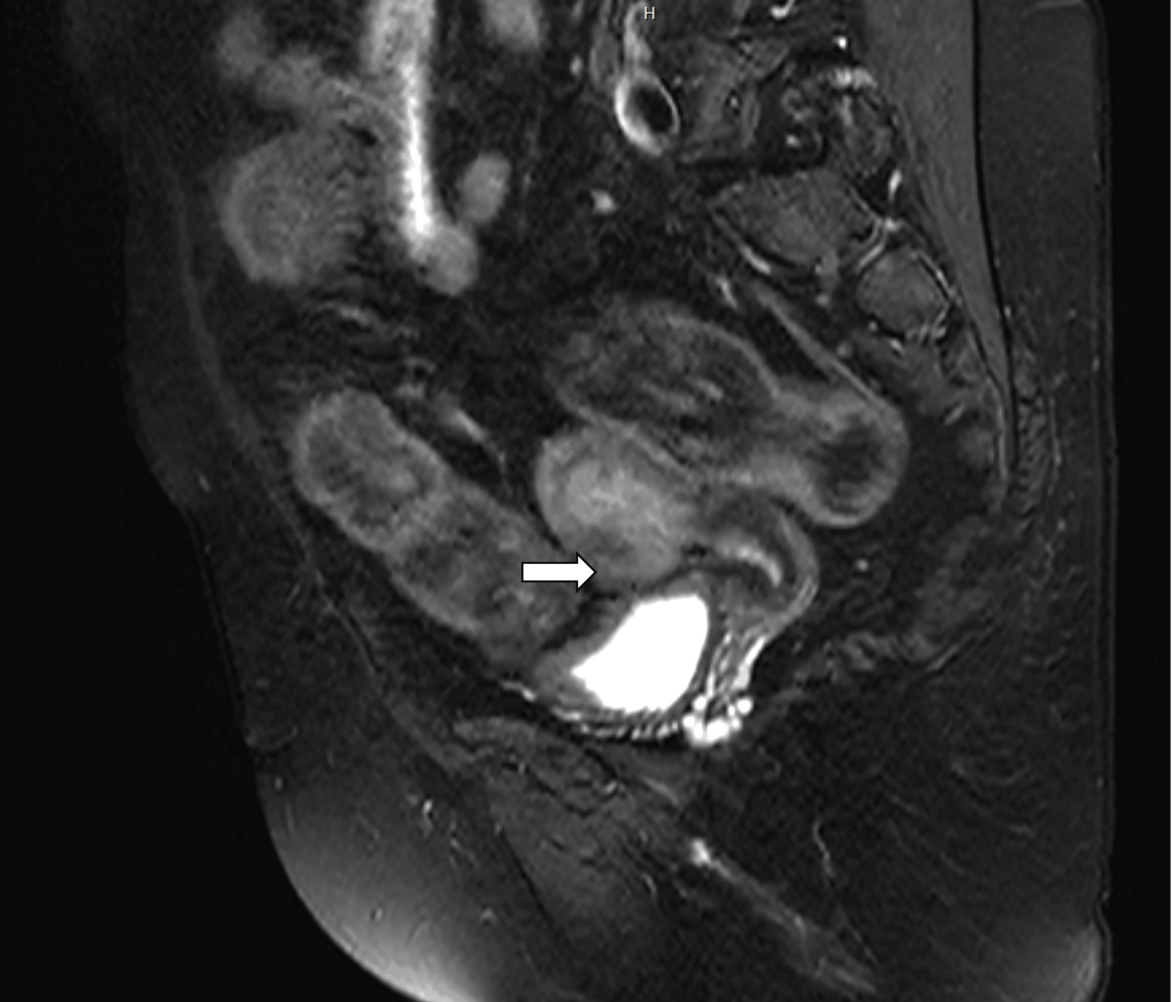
T2-weighted fast spin echo (FSE) SAG image shows an ill-defined, heterogeneously hyperintense endometrial mass (arrow) extending through the full thickness of the myometrium, with suspected serosal involvement. SAG – Sagittal plane
